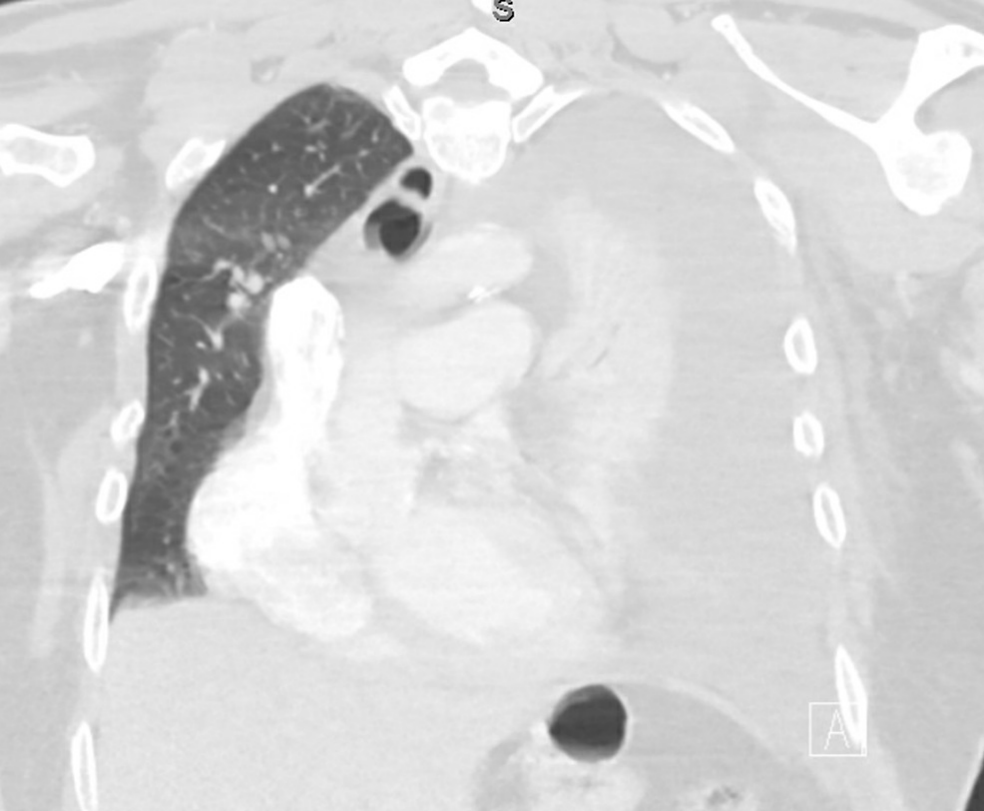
Coronal CT with evidence of large left hydrothorax with mediastinal shift
